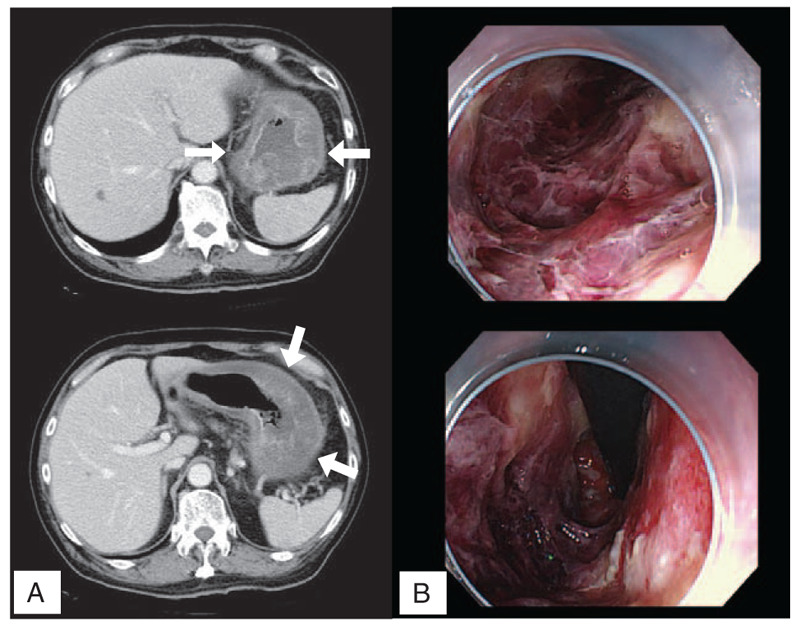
(A) Contrast-enhanced computed tomography image at the onset showing a diffuse thickened gastric wall (arrows). (B) Upper gastrointestinal endoscopy showing extensive gastric deep ulcerations with necrotic tissue and an edematous mucosa.
